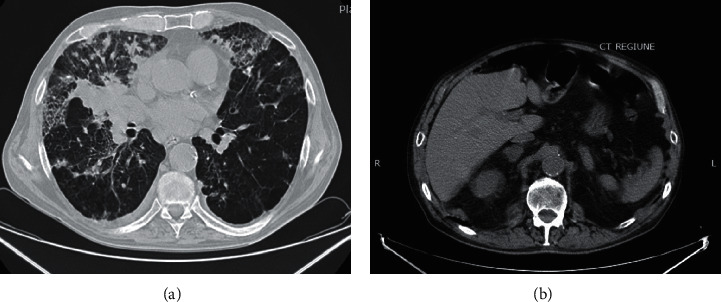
Thoracic CT at the initial diagnosis, without intravenous administration of contract material (increased creatinine levels): (a) dense mediastinal pulmonary mass localized in the medium lobe of the right lung, which meets the pulmonary hilum, and cannot be separated from the mediastinal structures, mediastinal, and retrocrural lymphadenopathies; (b) adrenal glands with diffuse increased sizes and spontaneous tissue density.
